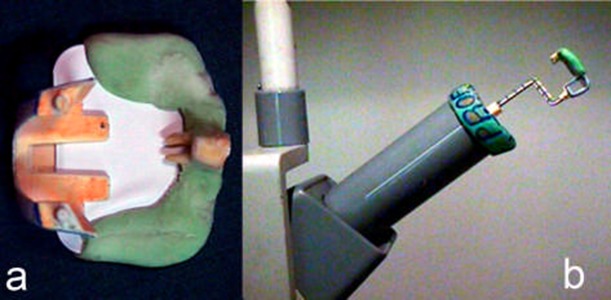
a) A radiograph mount for maintaining the tooth-film position constant, b) An Endo-ray attached to X-ray tube by acrylic resin
